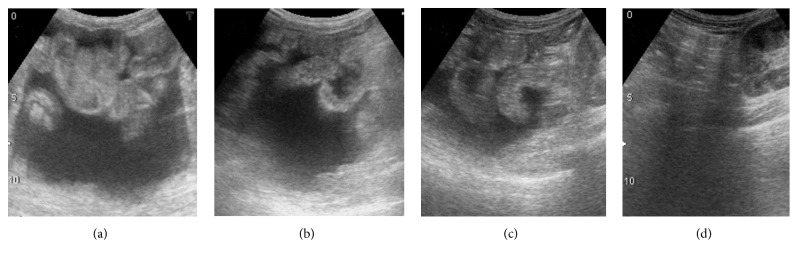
Lower abdominal ultrasonography. (a) Before percutaneous abdominal puncture; (b) 2 weeks after abdominal puncture; (c) 4 weeks after abdominal puncture; and (d) 6 weeks after abdominal puncture (ascites completely resolved).
